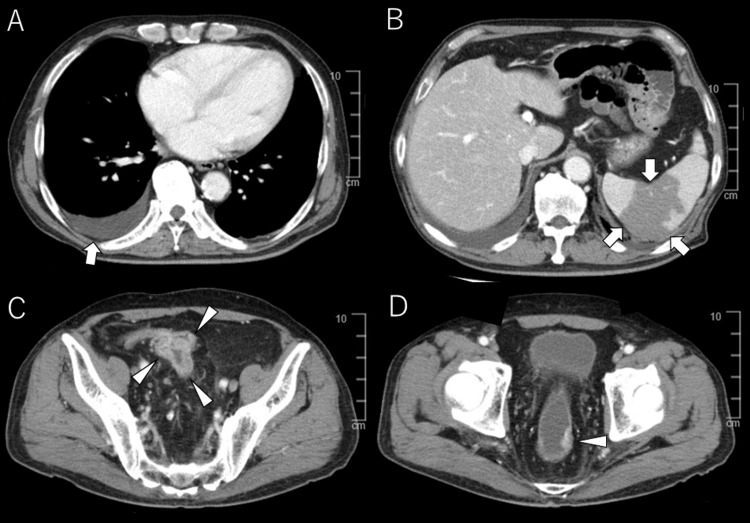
Abdominal contrast-enhanced CT. (A) Cardiomegaly with right-sided pleural effusion is observed (arrow). (B) An irregular hypodense area in the spleen is noted, suggestive of splenic infarction (arrow). (C) A localized wall thickening is observed in the sigmoid colon, with heterogeneous post-contrast enhancement, suggestive of sigmoid colon cancer (arrowhead). (D) A small protruding lesion is noted on the left wall of the rectum, consistent with rectal cancer (arrowhead).
